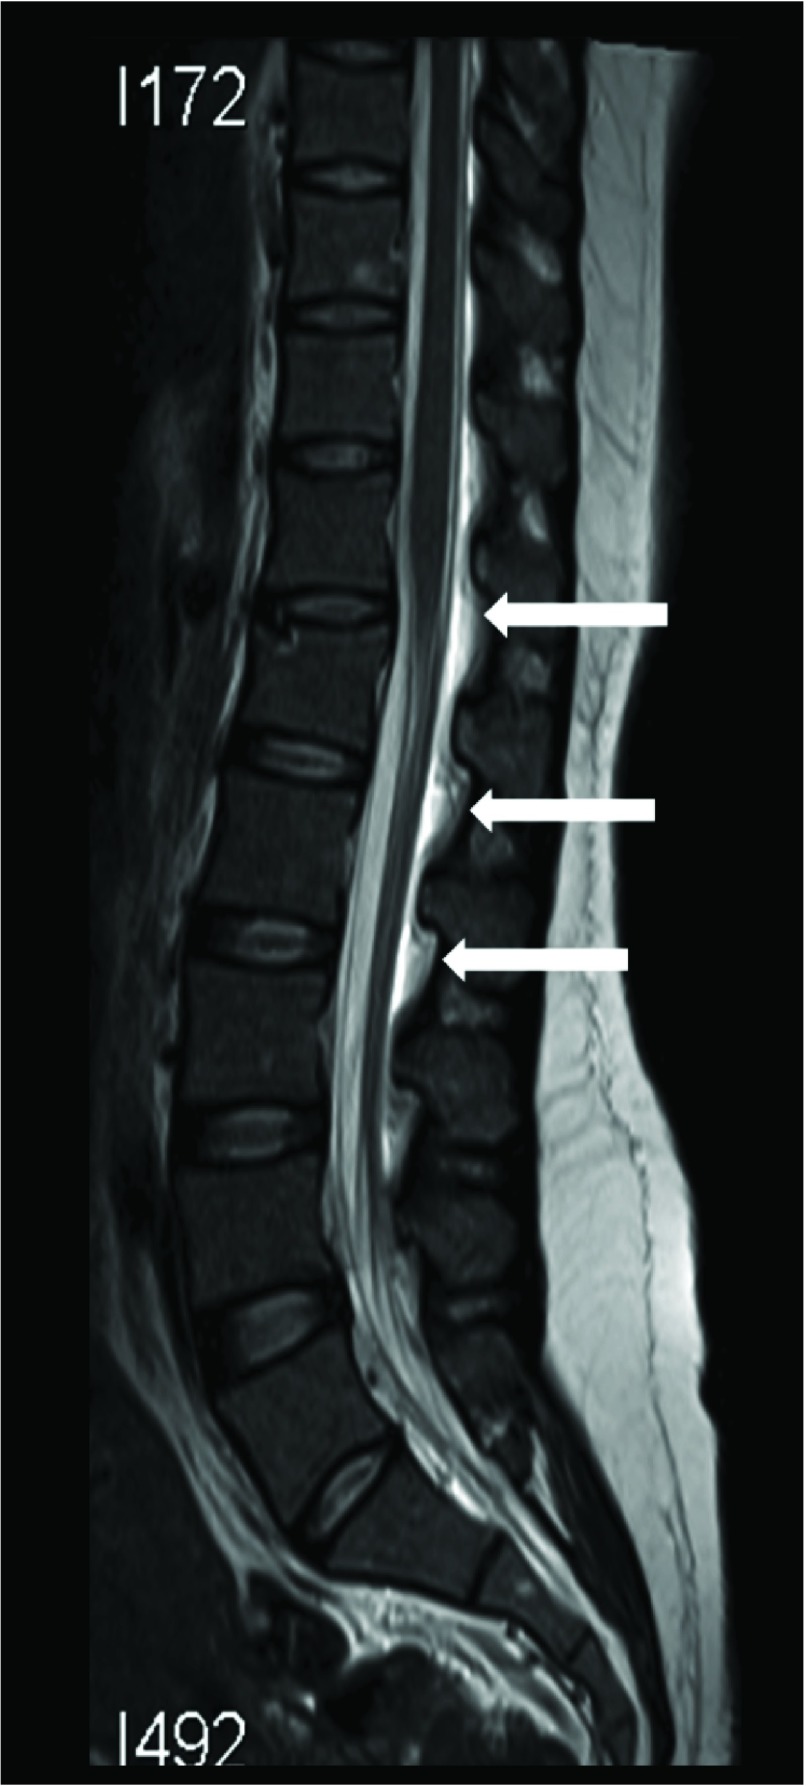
MR imaging of the spine. T2W sequence shows rim epidural CSF collection in the posterior aspect D11–L3 vertebra suggestive of CSF leak (white solid arrow).
